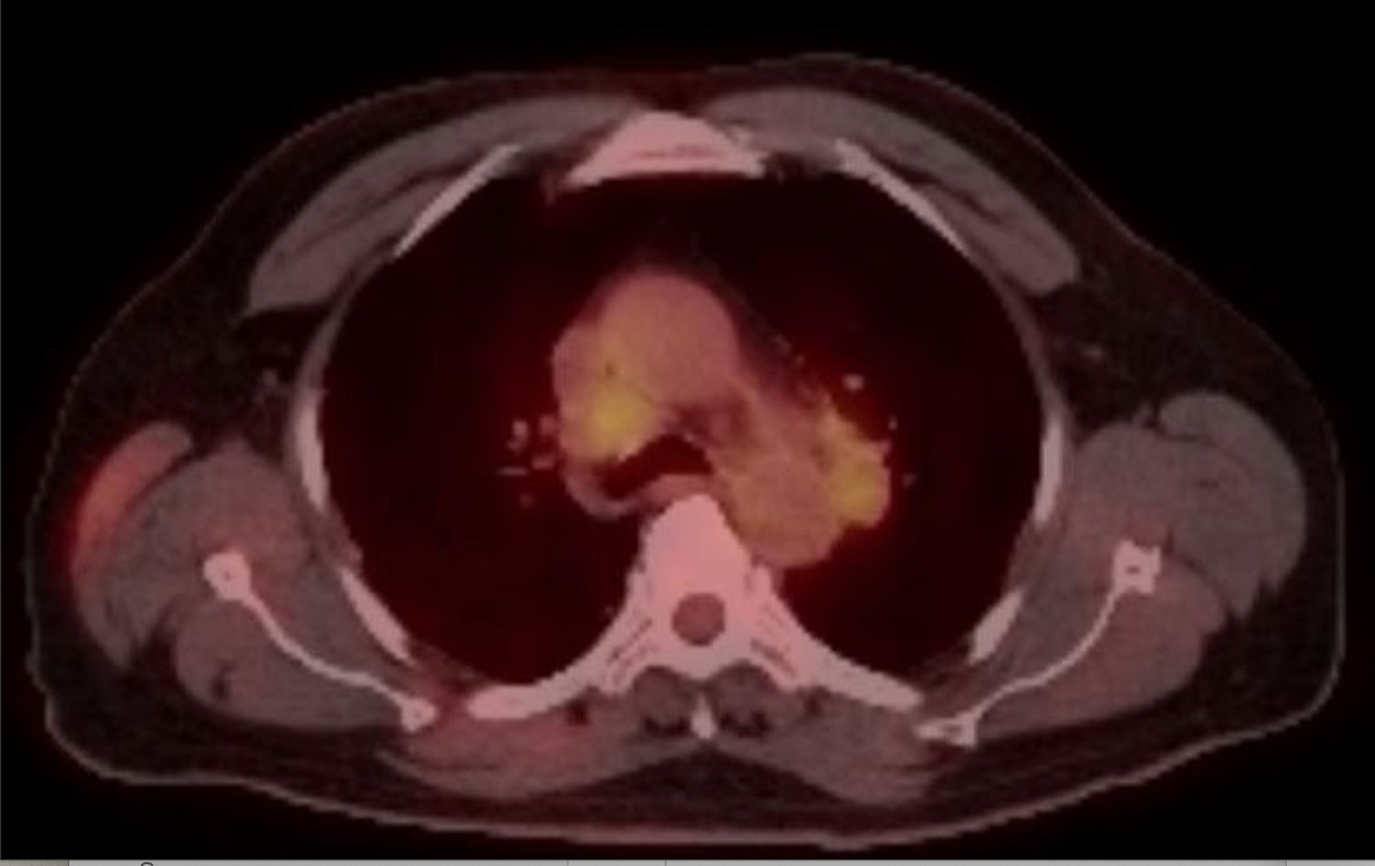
PET/CT showing hypermetabolic mediastinal lymph nodes in the precarinal and left prevascular spaces. PET/CT: positron emission computerized tomographic scanning.
